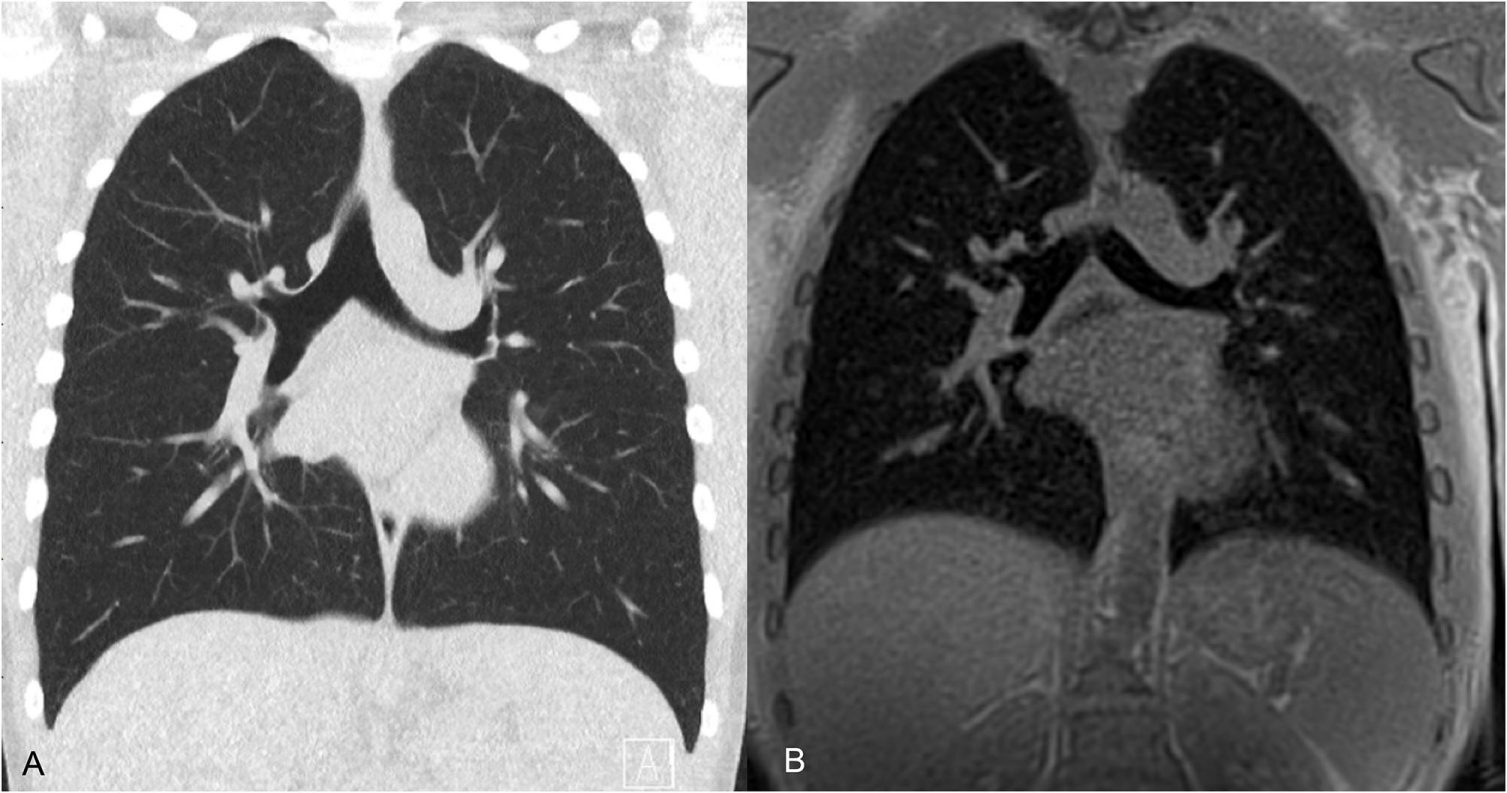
CT and MRI of the airways in a 12-year-old male. (A) Coronal 3-mm low dose CT acquired at total lung capacity shows bronchi to the fourth (subsegmental) airway generation. (B) MRI averaged over 3 min free breathing, therefore close to functional residual capacity, shows bronchi to the third (segmental) airway generation.
